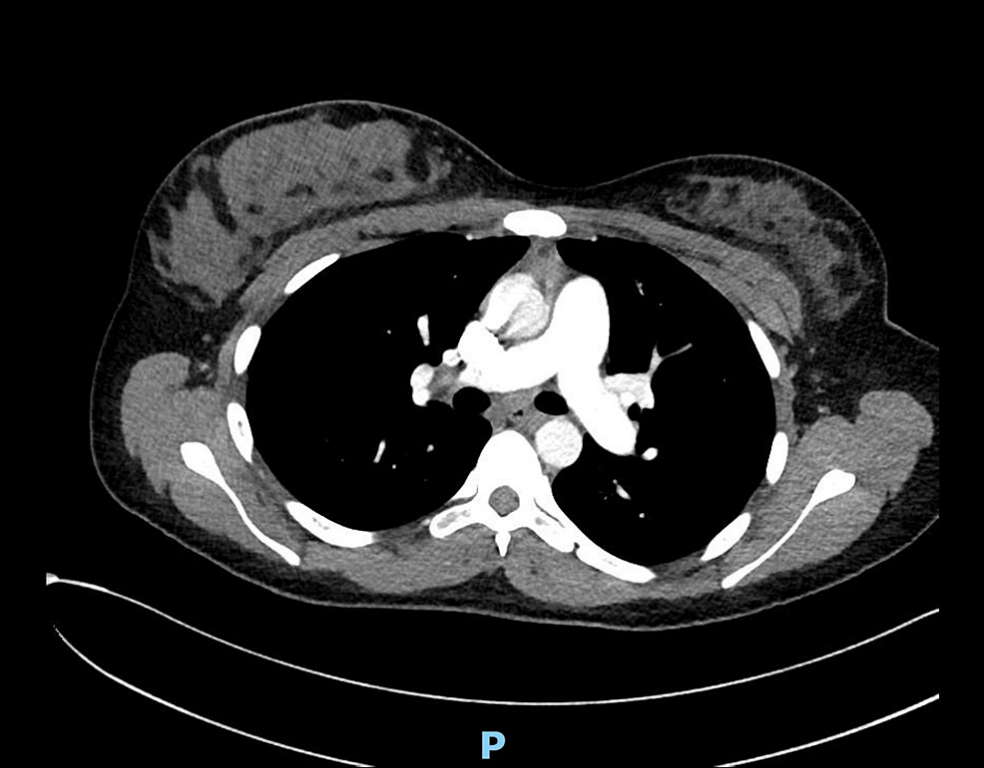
Computed tomography angiography showing no evidence of pulmonary embolism
